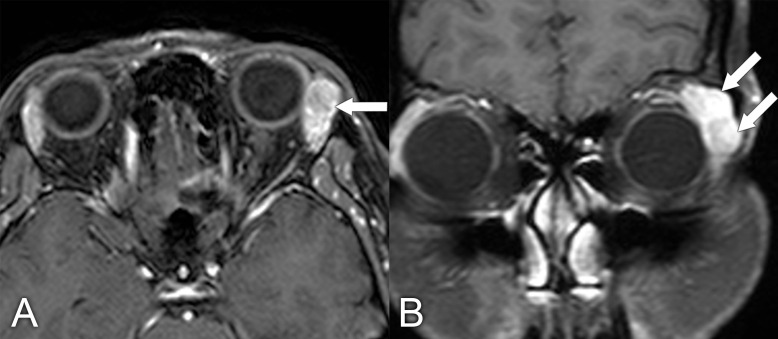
MRI findings in a 55-year-old woman who presented with early-stage (T2) lacrimal gland adenoid cystic carcinoma. (A) Axial T1 postcontrast MRI with fat suppression shows an elongated lesion with posterior extension that enhances with contrast (arrow). (B) Coronal T1 postcontrast MRI with fat suppression shows that the lesion is multilobular and abuts the superior rectus and lateral rectus muscles (arrows).
